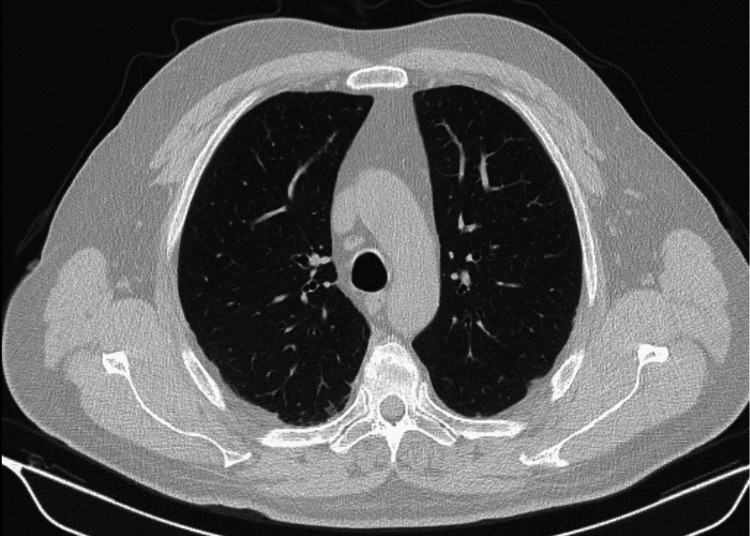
Chest CT reevaluation five months after diagnosis and initiation of corticosteroid therapy
